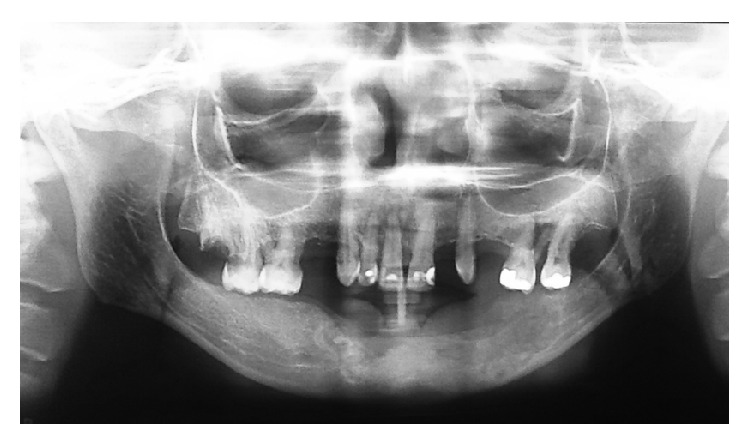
Panoramic radiograph 12 months after the surgery.
